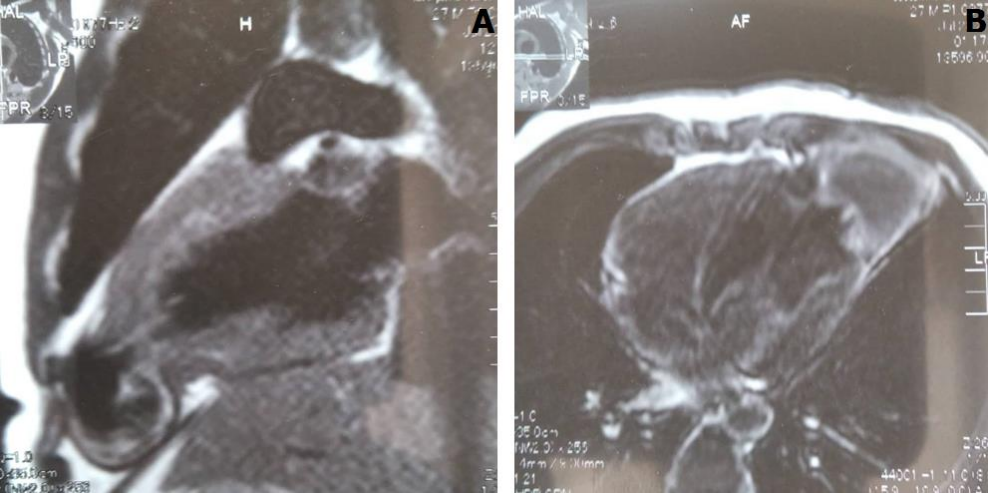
left apical congenital ventricular aneurysms at magnetic resonance imaging (A, B)
